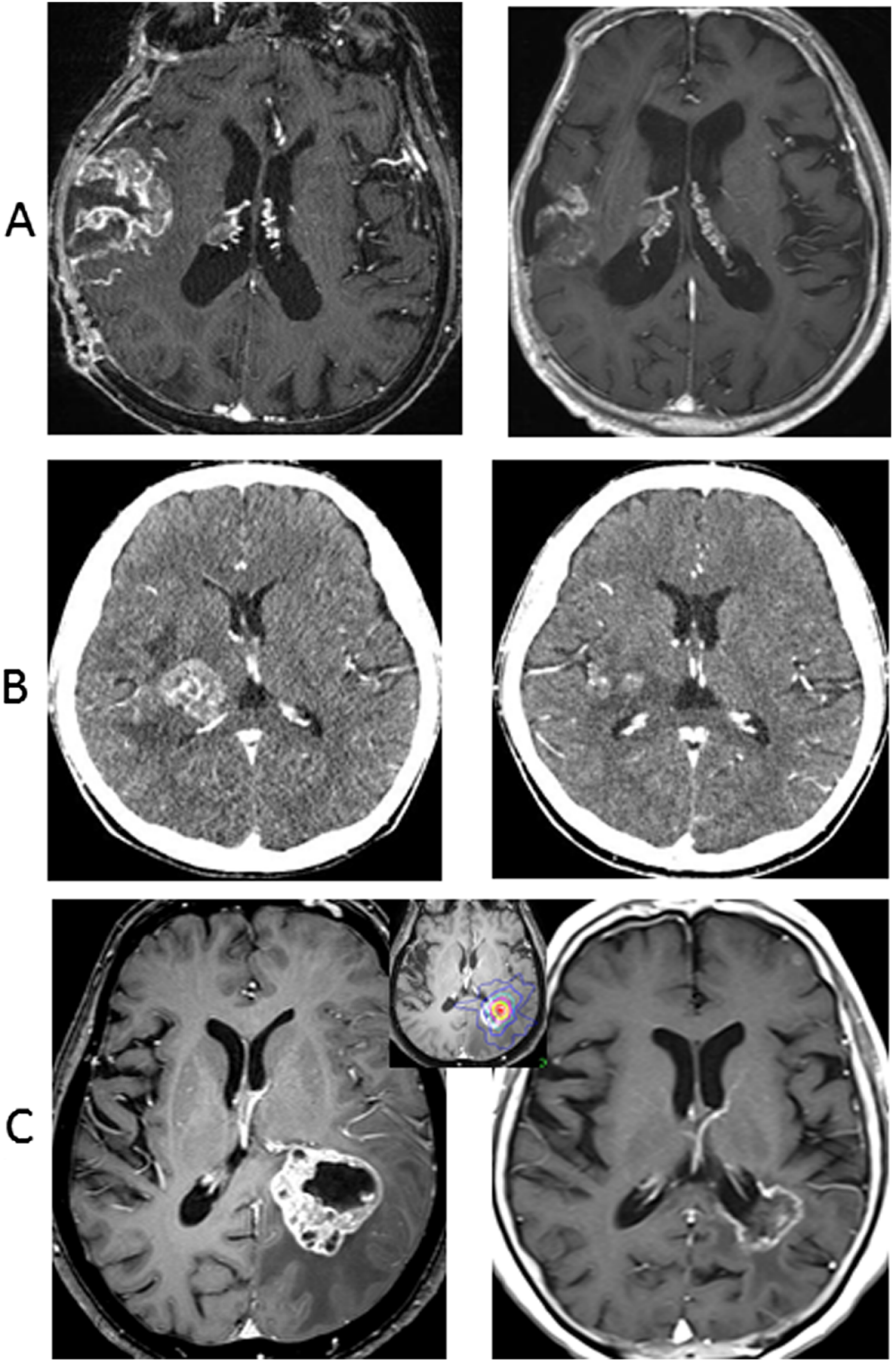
Tumor regression after optimal hypofractionated conformal radiotherapy. A: Gd-enhanced T1-weighted MR images. Lung cancer brain metastasis in a 73-year-old male. A large residual tumor (74.6 cm^3^) obtained after partial removal due to an impending brain hernia was treated with a marginal dose of 27 Gy in five fractions at an isodose of 56% (left). A significant tumor response with no adverse imaging effects was found four months after the administration of conformal radiotherapy (right). The patients’ left hemi-paresis disappeared, and the KPS improved from 60 to 70. B: Contrast-enhanced CT scans (MR images not available for the pace maker implant). Lung cancer brain metastasis in a 52-year-old female. A tumor in the thalamus (10.6 cm^3^) with perifocal edema was treated with a marginal dose of 31 Gy in five fractions at an isodose of 63% (left). A tumor response was observed seven months after the administration of conformal radiotherapy (right). The patients’ left hemiparesis was ameliorated, and the KPS improved 60 to 70 (walking with a stick 32 months after treatment). C: Gd-enhanced T1-weighted MR images. Breast cancer brain metastasis in a 70-year-old female. A large tumor in the parietal lobe (23.5 cm^3^) with perifocal edema was treated with a marginal dose of 35 Gy in eight fractions at an isodose of 57% (left). A tumor response was found two months after treatment, Gerstmann’s syndrome disappeared, and the KPS improved 60 to 70. Marginal recurrence was noted 20 months after the first treatment, and the recurrent lesion (1.7 cm^3^) was treated with a marginal dose of 20 Gy at an isodose of 69% in single-session radiosurgery (center). A tumor response with no adverse imaging effects was found 34 months after the first treatment (right).
